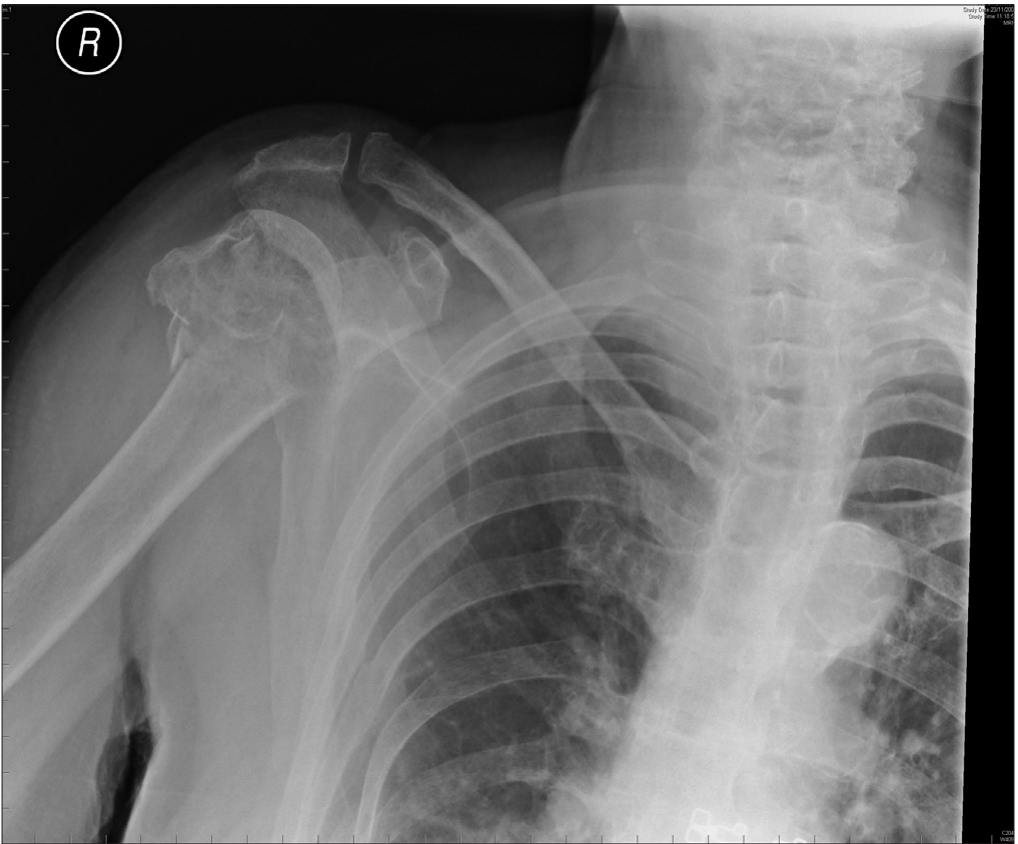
Plain AP radiograph of right shoulder. A ‘Neer’ 2-part fracture is seen
